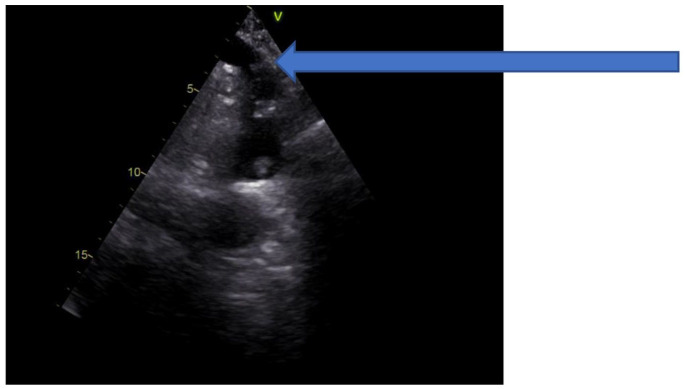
Transthoracic echocardiogram presenting unclear floating structure in ascending aorta (arrow).
